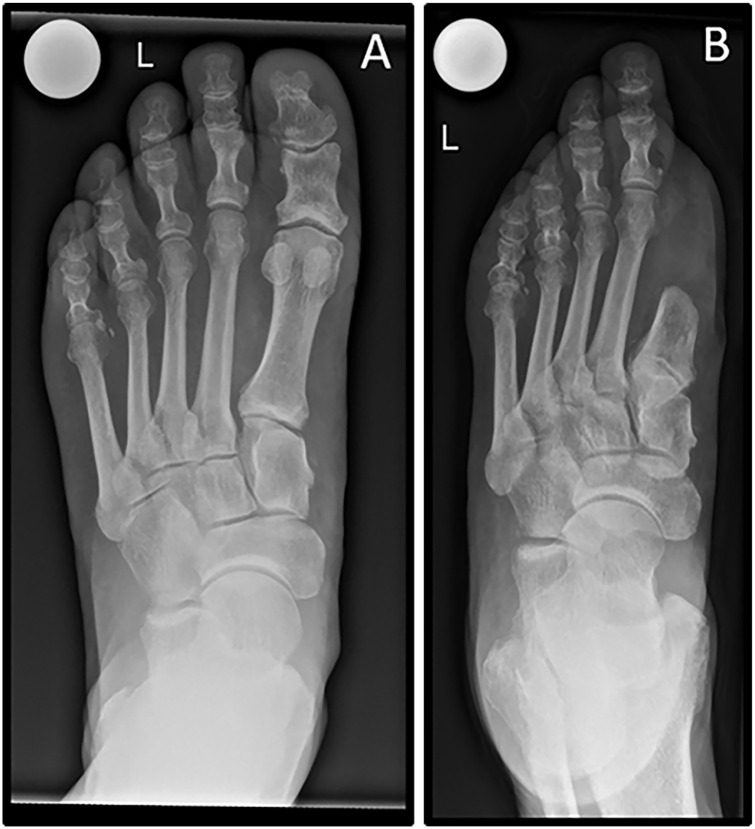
(A) A radiograph of the left foot (dorsoplantar view, weightbearing) of a patient who is to undergo first-ray amputation. (B) Radiograph of the same left foot (dorsoplantar view, nonweightbearing) 2 months after first-ray amputation.
